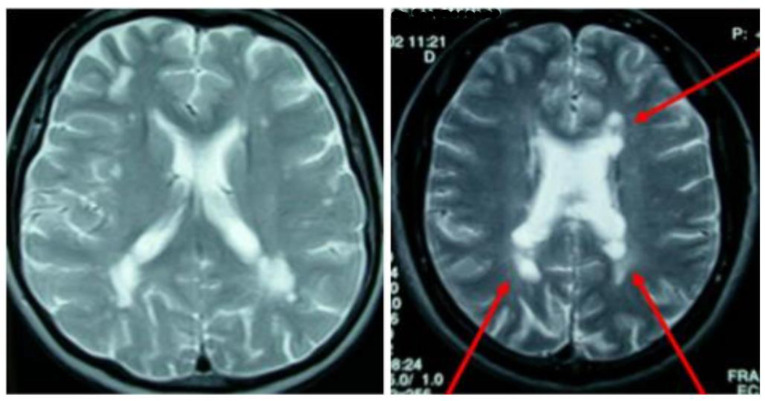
Periventricular lesions on MRI.
